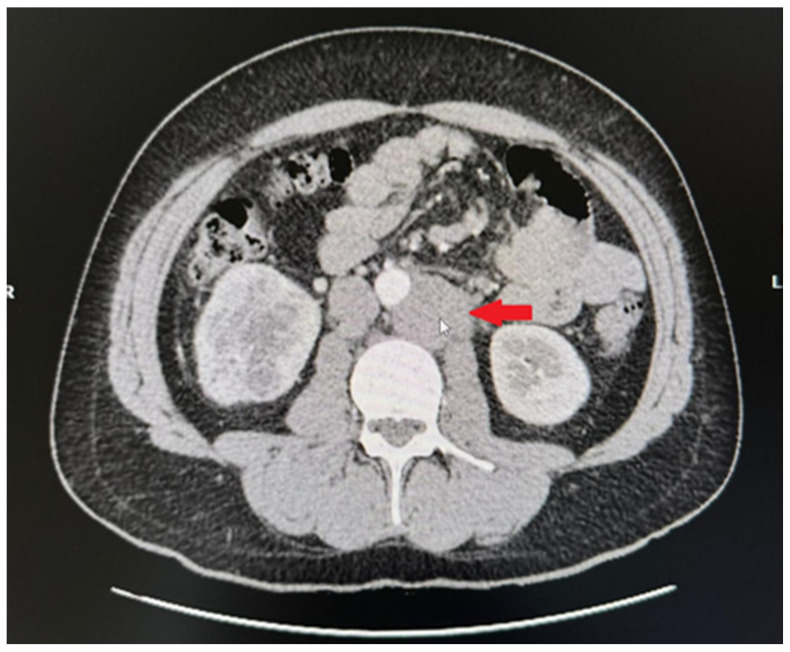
CT image. Pathological paraaortic and paracaval lymph nodes.
